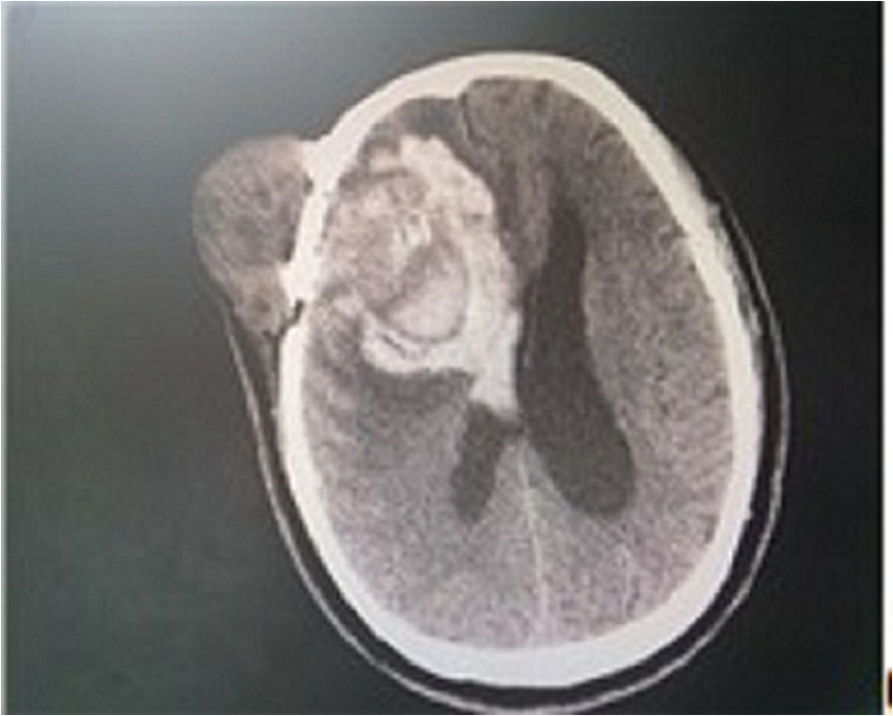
Preoperative computed tomography. Shows intracranial hemorrhage with a volume of approximately 90mL, and a midline shift of over 1.5cm.
